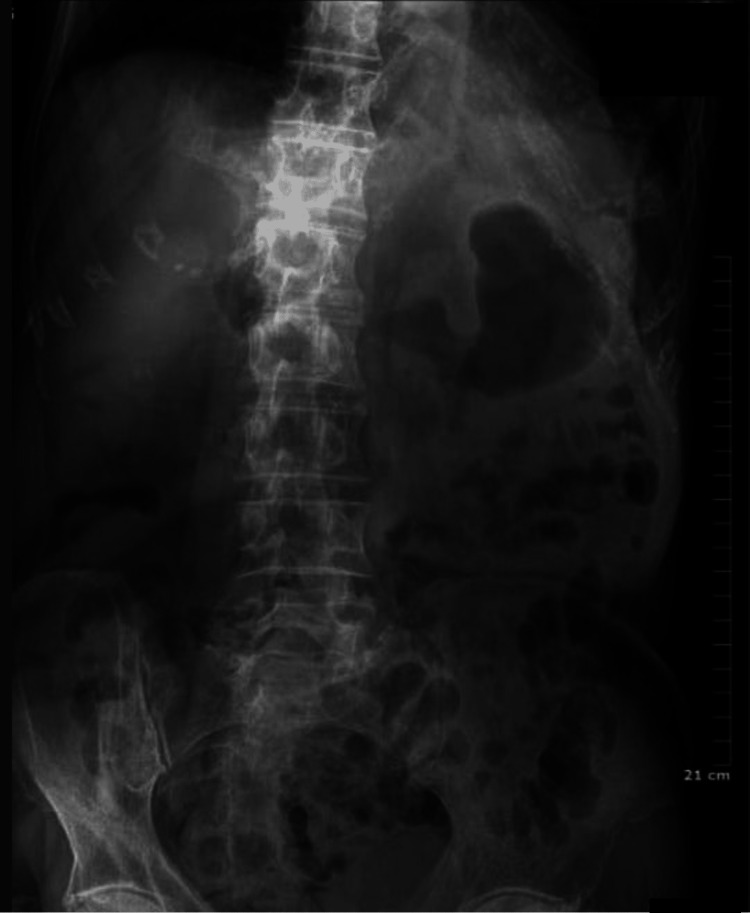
Abdominal X-ray showing fecal matter in colon
